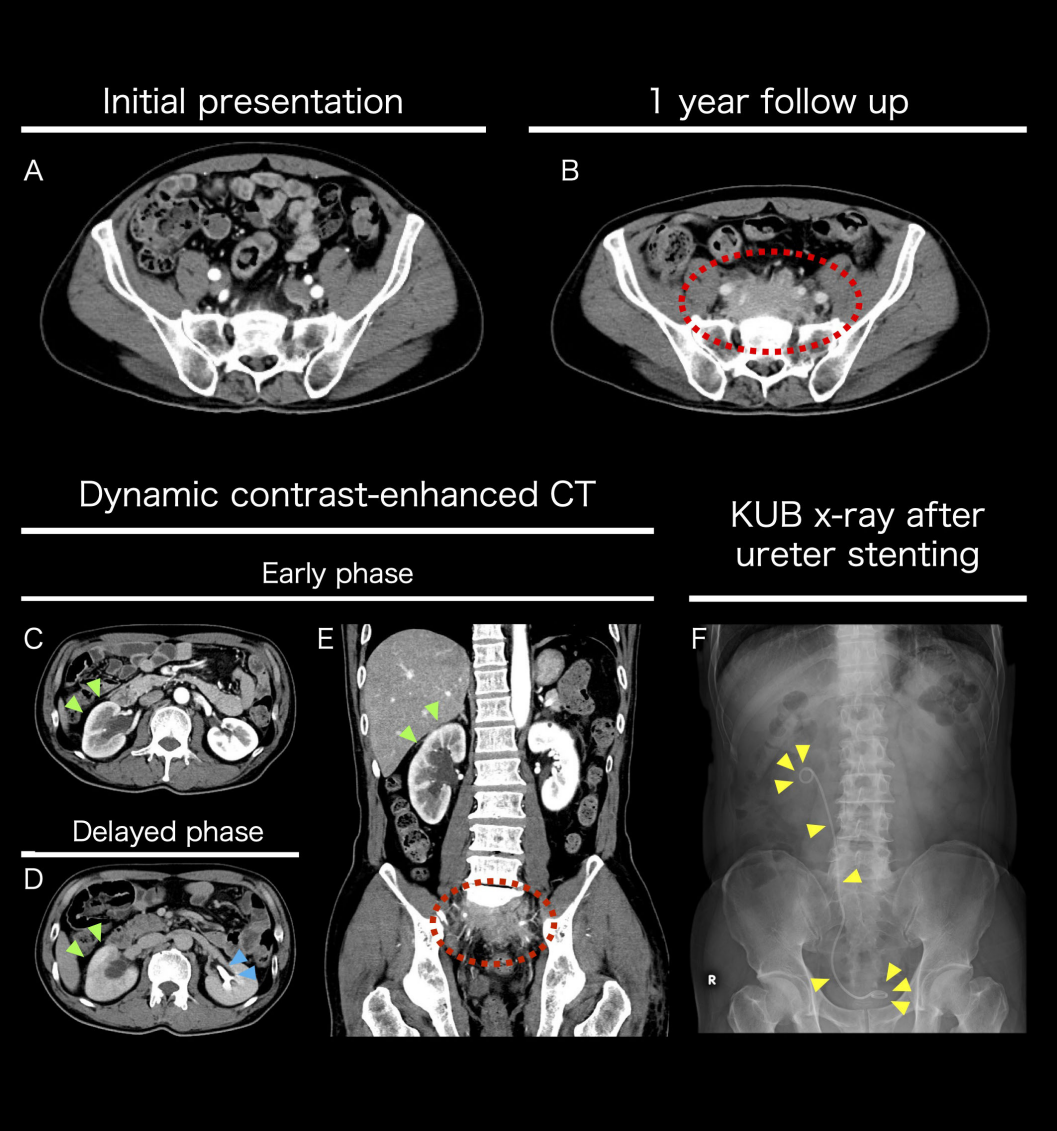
Imaging studies performed pre-hospital admission (A-F) Axial contrast-enhanced CT images at the sacral S1 level. Comparative images of the patient at the initial visit (A) and after one year (B). A growing mass was observed anterior to the sacrum. Axial dynamic contrast-enhanced CT images at the renal hilum (L2 level). Comparison between the early phase (C) and the delayed phase (D). The yellow-green arrow indicates poor contrast enhancement of the right renal cortex, suggesting reduced urine production. The light blue arrow indicates normal function of the left kidney, with urine being produced in the renal pelvis during the delayed phase. Coronal dynamic contrast-enhanced CT image in the early phase (E). The yellow-green arrow indicates reduced contrast enhancement of the right renal cortex, while the dashed red circle marks the mass. The mass caused right ureteral stenosis, leading to hydronephrosis. To preserve renal function, a right ureteral stent was placed transurethrally. Post-stenting KUB X-ray image (F).
